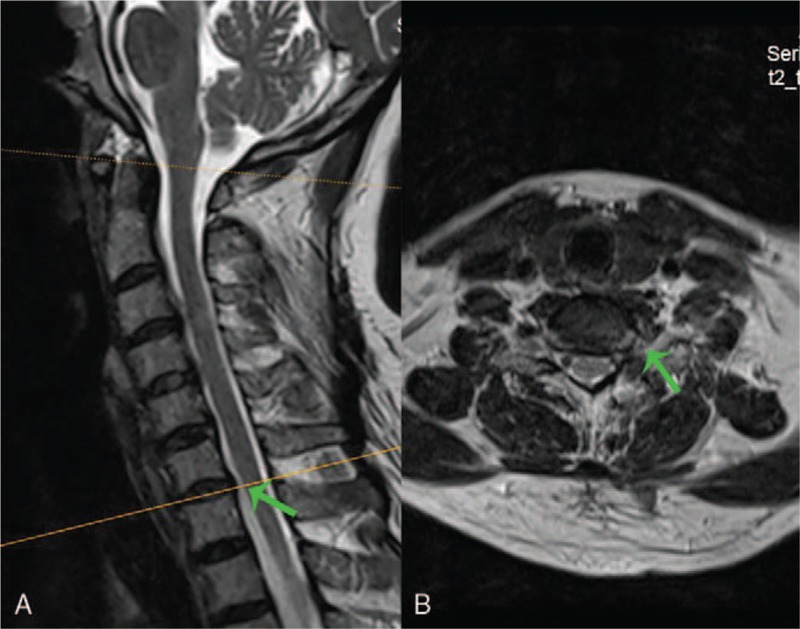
An magnetic resonance imaging examination was performed 1 week after the operation. (A) Sagittal images showed that the dual sac compression at the C6-7 segment was relieved (green arrow), (B) and axial images showed no compression at the left outlet nerve root of C6-7 (green arrow).
